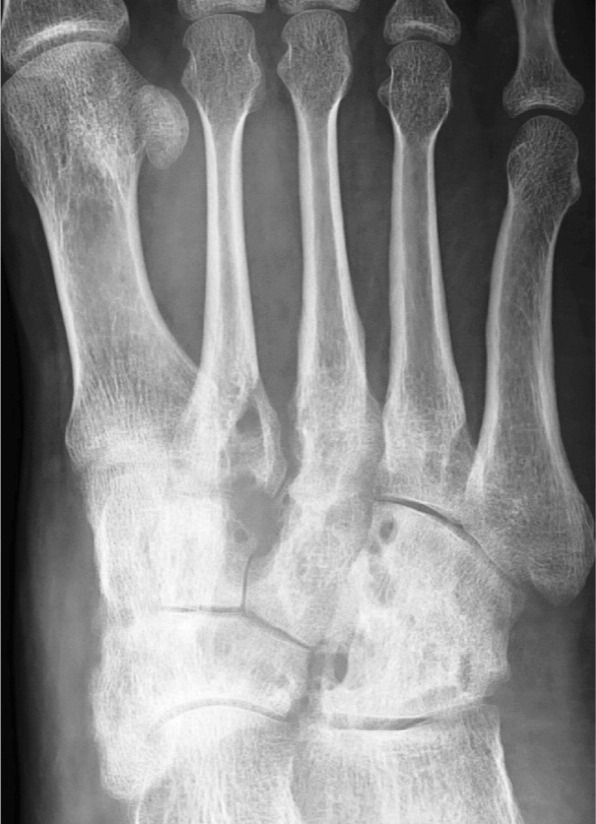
Pre operative radiograph of the foot showing multiple lytic lesions involving the mid foot bones and joints.
